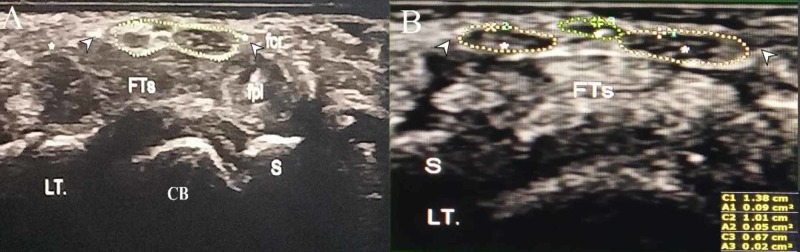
High-frequency linear transverse musculoskeletal ultrasonogram scan of left volar wrist. (A) transverse ultrasonogram of hypoechoic trifid median nerve segments encircled within hyperechoic sheath (dotted line). (B) trifid median nerve diameters (asterisks within dotted lines) for small, medium and large segments were 6.7 mm, 10.1 mm, and 13.8 mm, respectively. Arrowheads, flexor retinaculum; FTs, flexor tendons; CB, carpal bone; fcr, flexor carpi radialis tendon; fpl, flexor pollicis longus tendon; S, scaphoid; LT., left
